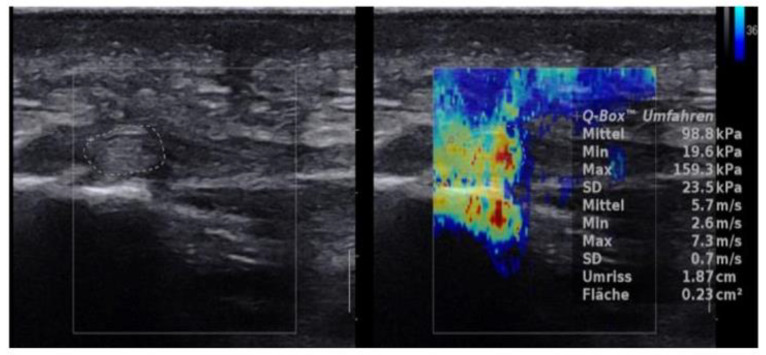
Longitudinal SWE image and B-mode ultrasound image of a 50-year-old patient with normal plantar fascia demonstrates stiffness of 98.8 kPa and SWV value of 5.7 m/s.
